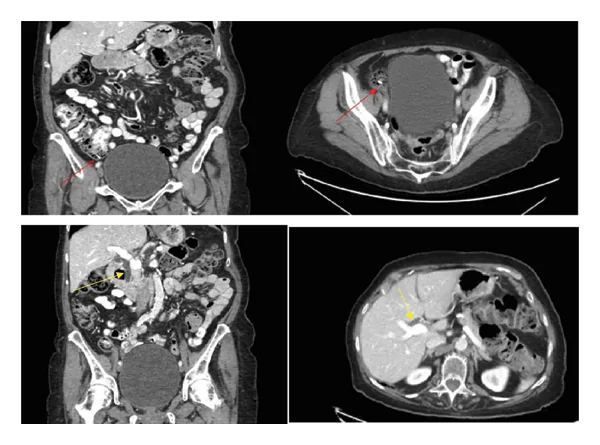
Computed tomography (coronal and axial views demonstrating clip location and CBD) 3 days after admission showing CBD dilation to approximately 10 mm (yellow arrows); previous intrahepatic bile duct distention is essentially resolved. The previously seen surgical clip in the region of the distal common bile duct is no longer evident, and there is a new clip in the region of the cecum (red arrows), presumably the migrated clip.
